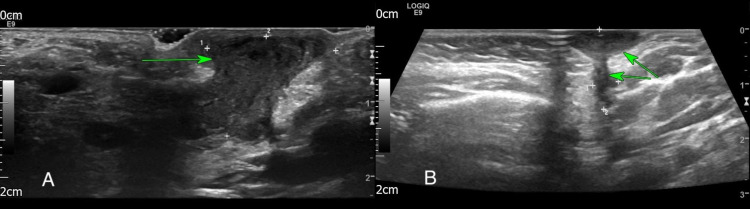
A: Ultrasound of the left neck showing debris subcutaneously adjacent to left parotid. B: Ultrasound of the left neck showing lenticular shaped collection with a hypoechoic tract extending from the superficial lesion into the posterior aspect of tail of left parotid salivary gland
